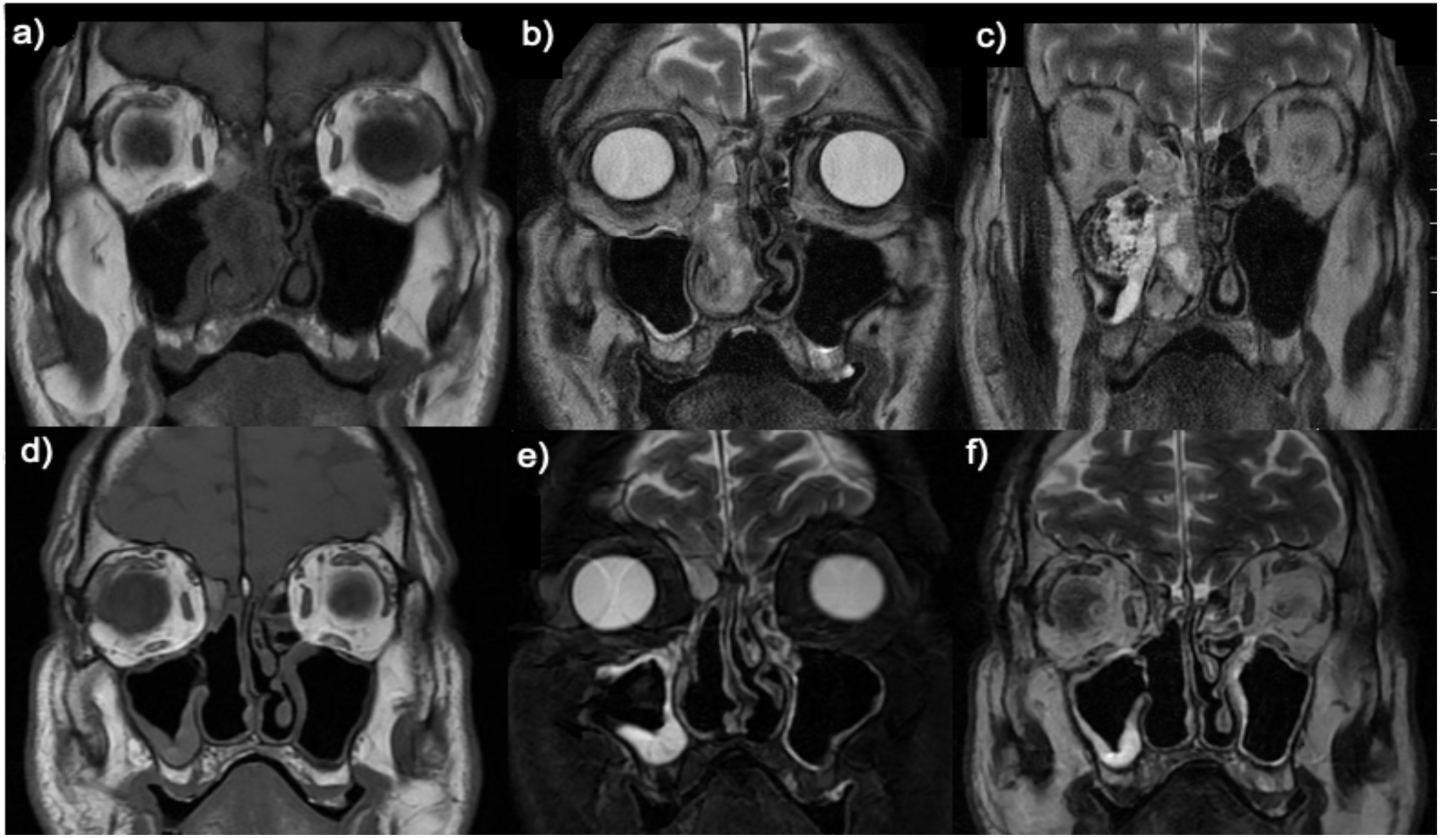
Preoperative (A–C) and postoperative (D–F) coronal T1 (A, D) and T2 (B, C, E, F) MRI images.
